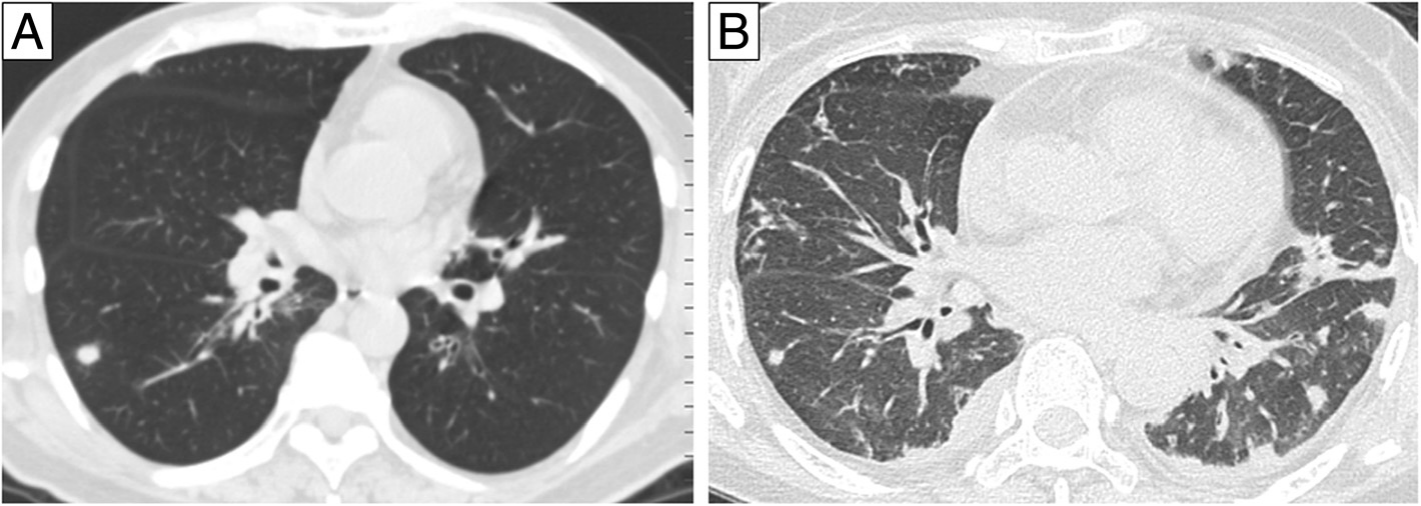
Representative photographs of computed tomography (CT) scans. a Initial CT scan of 63 y.o. male positive for PR3-ANCA shows a solitary nodule and wall thickning of lobar bronchi. b Initial high-resolution CT scan of 66 y.o female positive for MPO-ANCA shows several nodules, ground glass opacity, wall thickning of lobar and segmental bronchi, and pleural effusion
